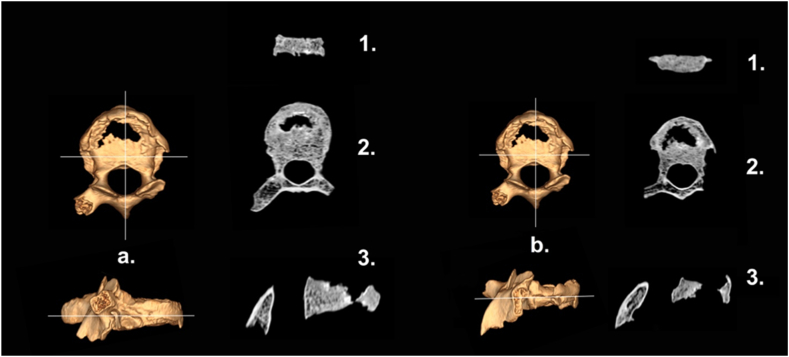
CT orthogonal sections of T5 (a.) & T8 (b.) 3D reconstruction; 1. coronal, 2) axial, 3) sagittal.
